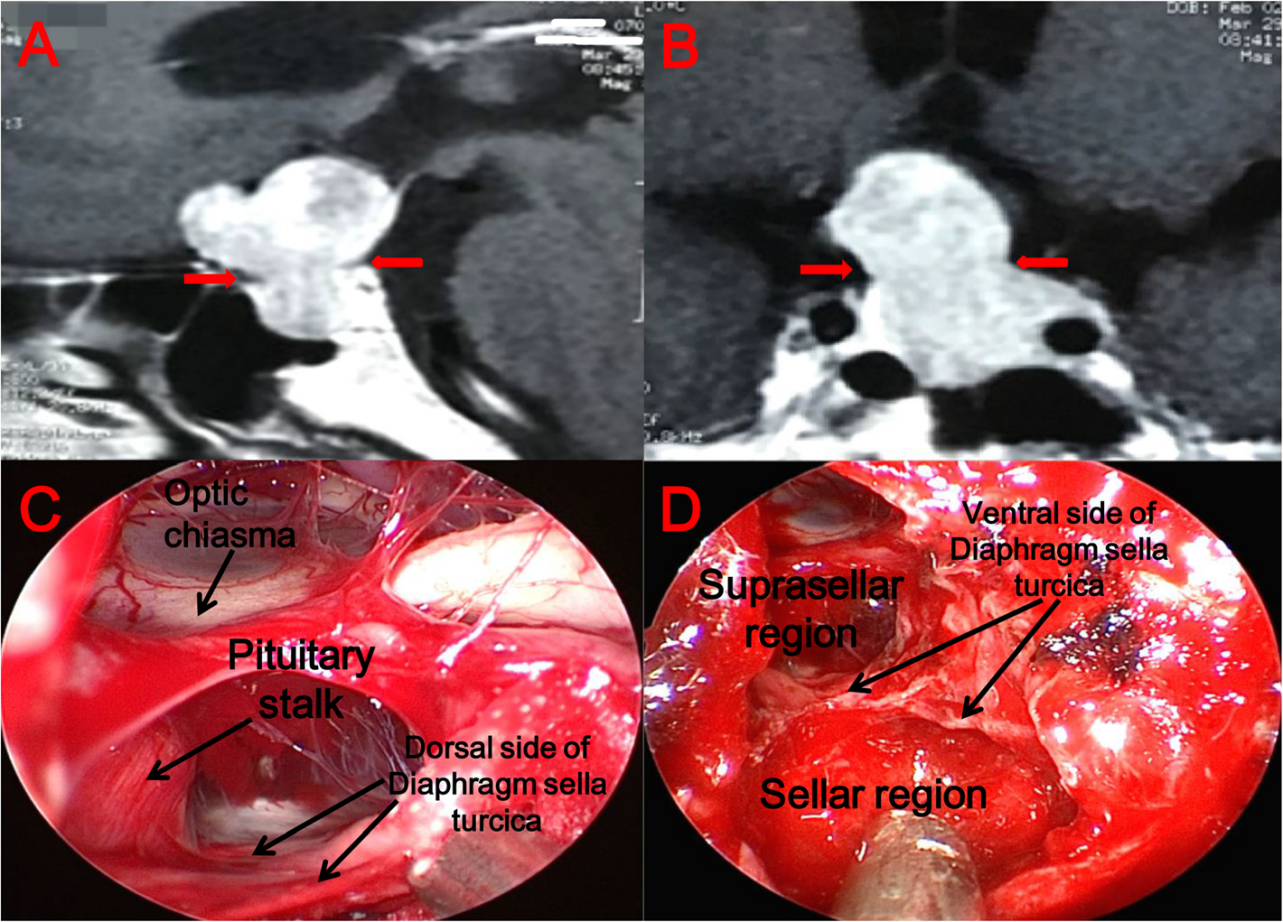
A patient with non-functional pituitary macroadenoma. (a, b) Sagittal and coronal views on MRI. The area pointed to by the red haircut is the place where the tumor is bound by the Diaphragm sella turcica. (c, d) These are the images during the operation, and we can see clearly the existence of Sellar diaphragm
